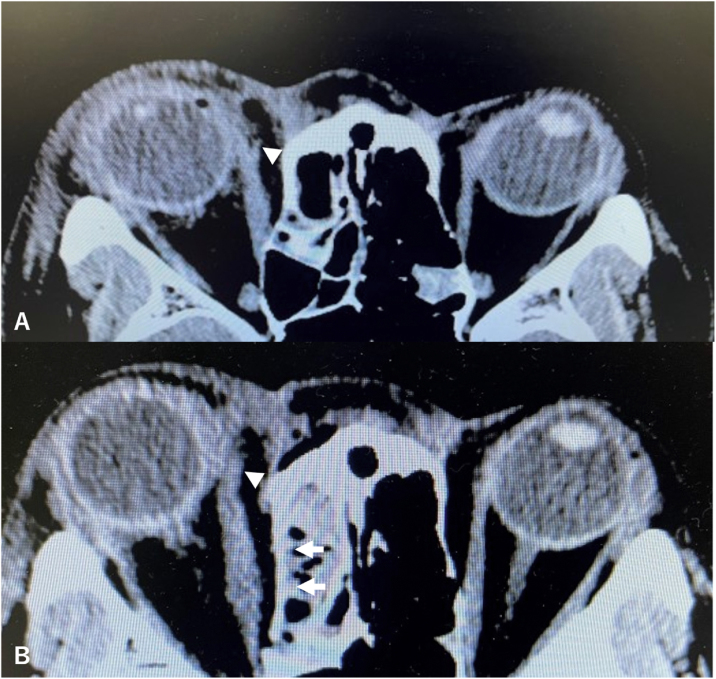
A, Preoperative axial Computed Tomography (CT) of the orbit showing transection of the medial rectus muscle. Arrowheads indicate the site of transection. B, Postoperative axial CT of the orbit showing retrieval of the medial rectus muscle. Arrowheads indicate the reconstructed medial rectus muscle. Arrows indicate the reconstructed medial wall of the orbit.
